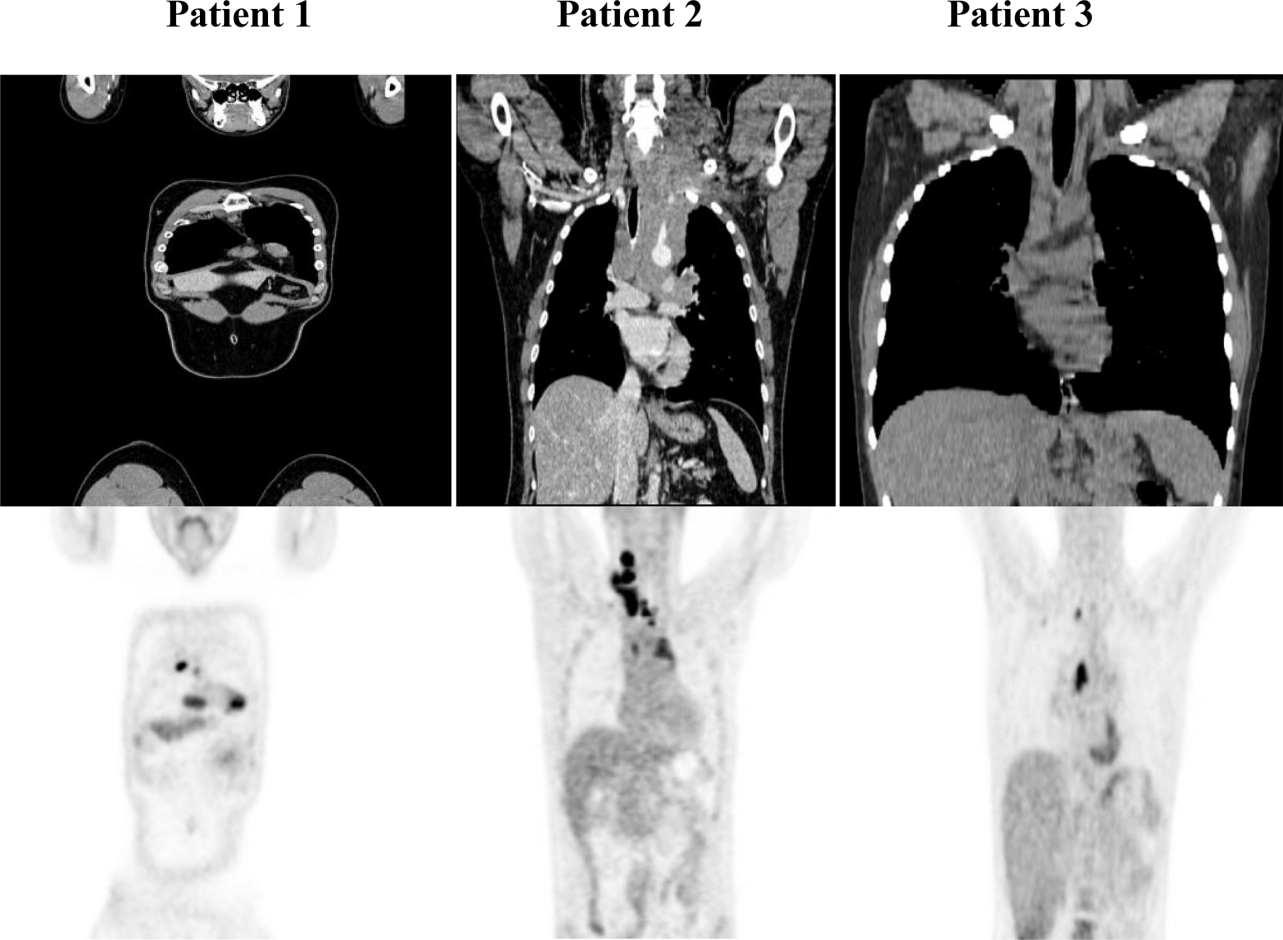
PET/CT of r/r cHL patients prior to the initiation of anti-PD-1 treatment. Patient 1 presented with conglomerate lymph node masses at the anterosuperior mediastinum with the size of 37x21 mm, SUV 6.4 and the paragastric conglomerate with the size of 45x42 mm, SUV 7.4. Patient 2 presented with cervical lymph nodes with the size of 6.6mm, SUV 4.7 and with conglomerate lymph node mass at the anterosuperior mediastinum with the size of 74x42 mm, SUV 7.7. Patient 3 presented with conglomerate lymph node mass at the anterosuperior mediastinum with the size of 43x32 mm, SUV 8.2. SUV, standardized uptake value.
